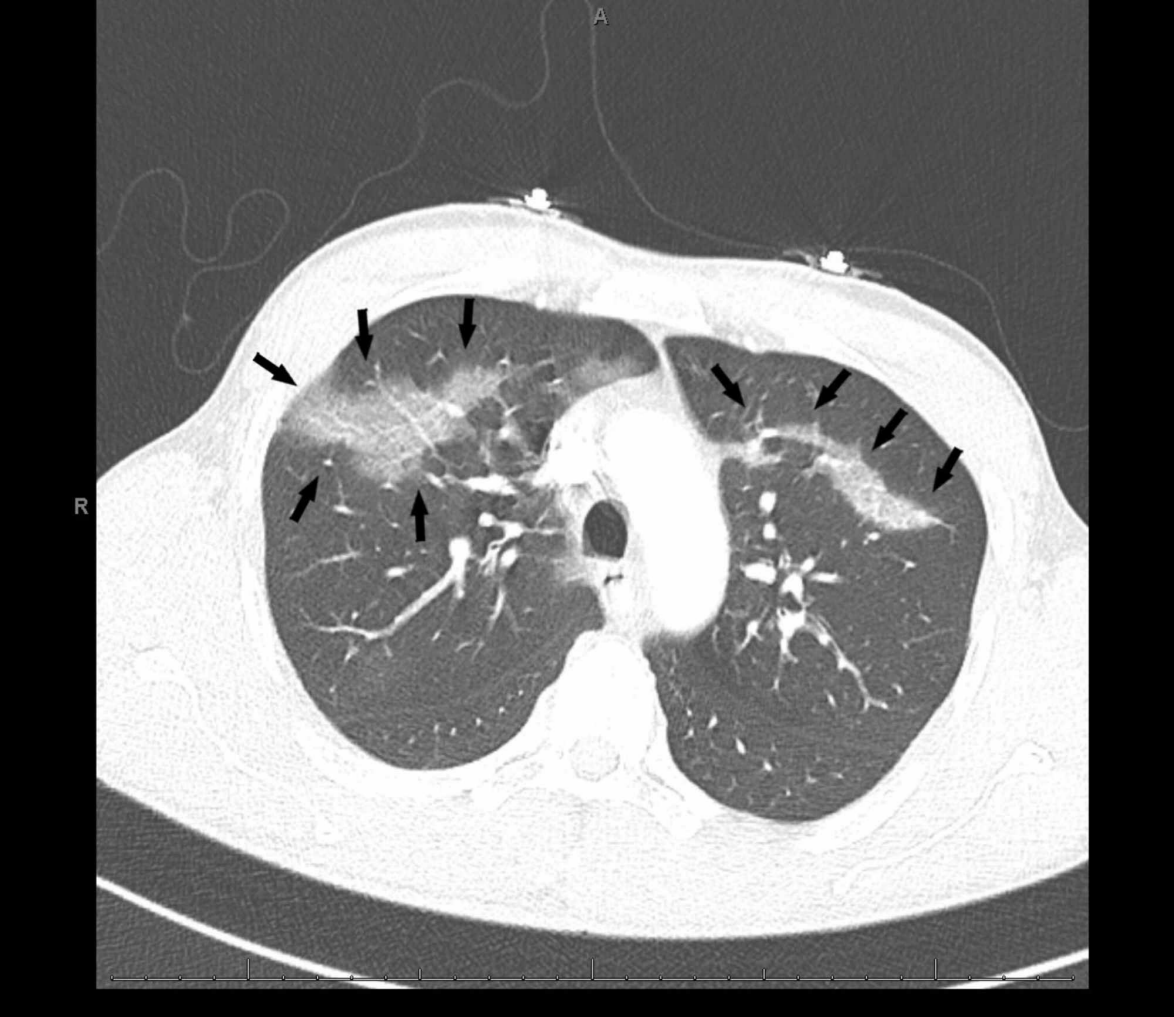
A computed tomography (CT) of the chest with bilateral ground-glass opacities (black arrows)
